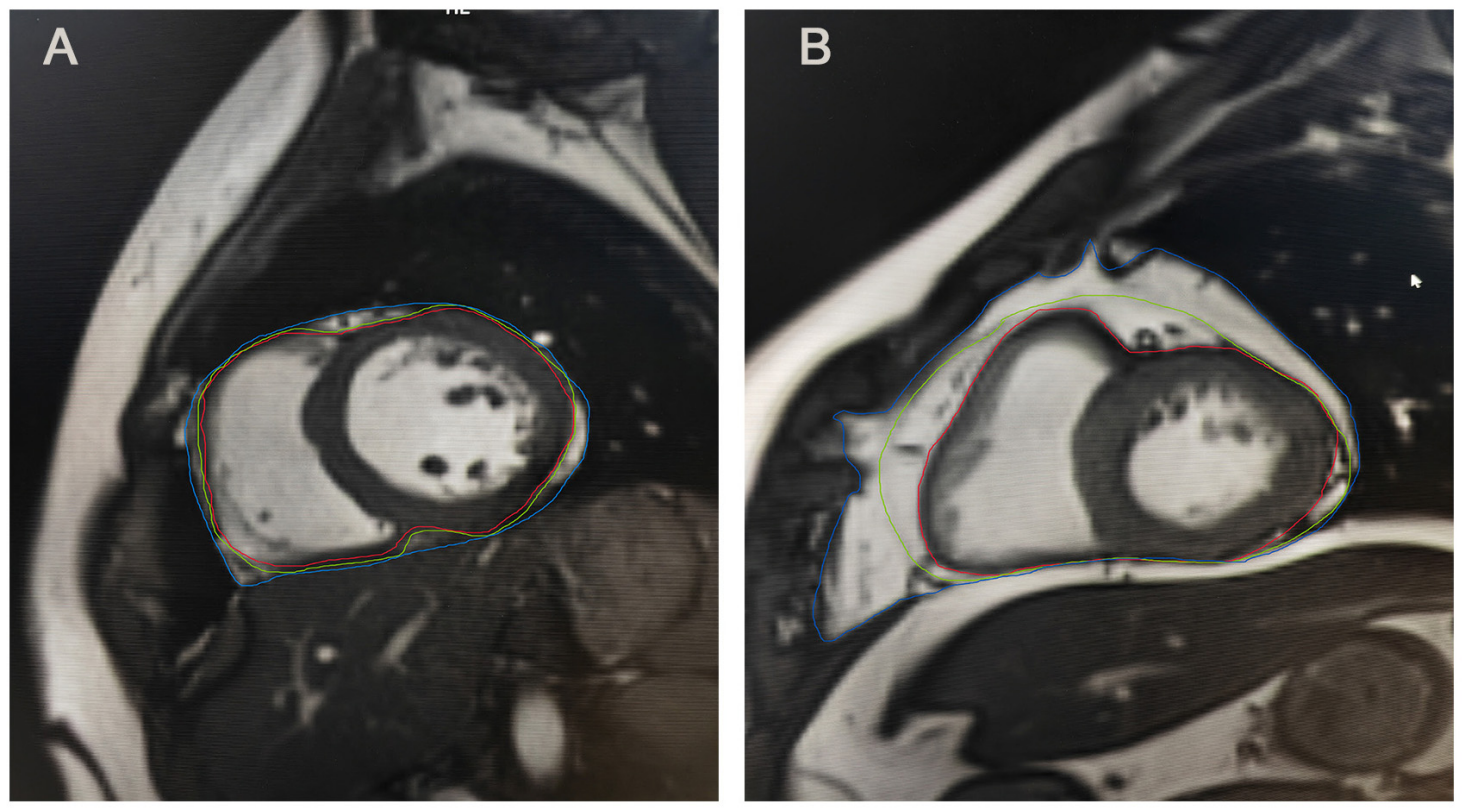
Epicardial and pericardial adipose tissue on MRI. (A)
